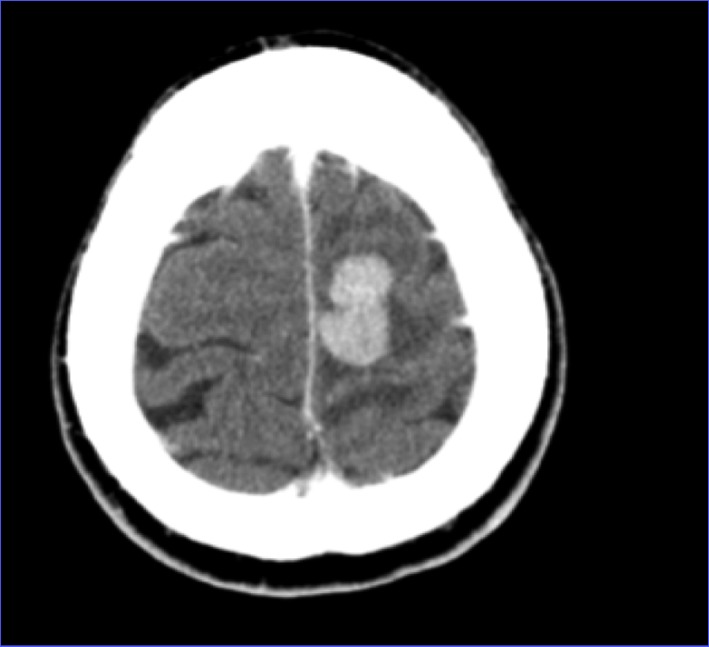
CT of head axial view showing left frontal mass.
